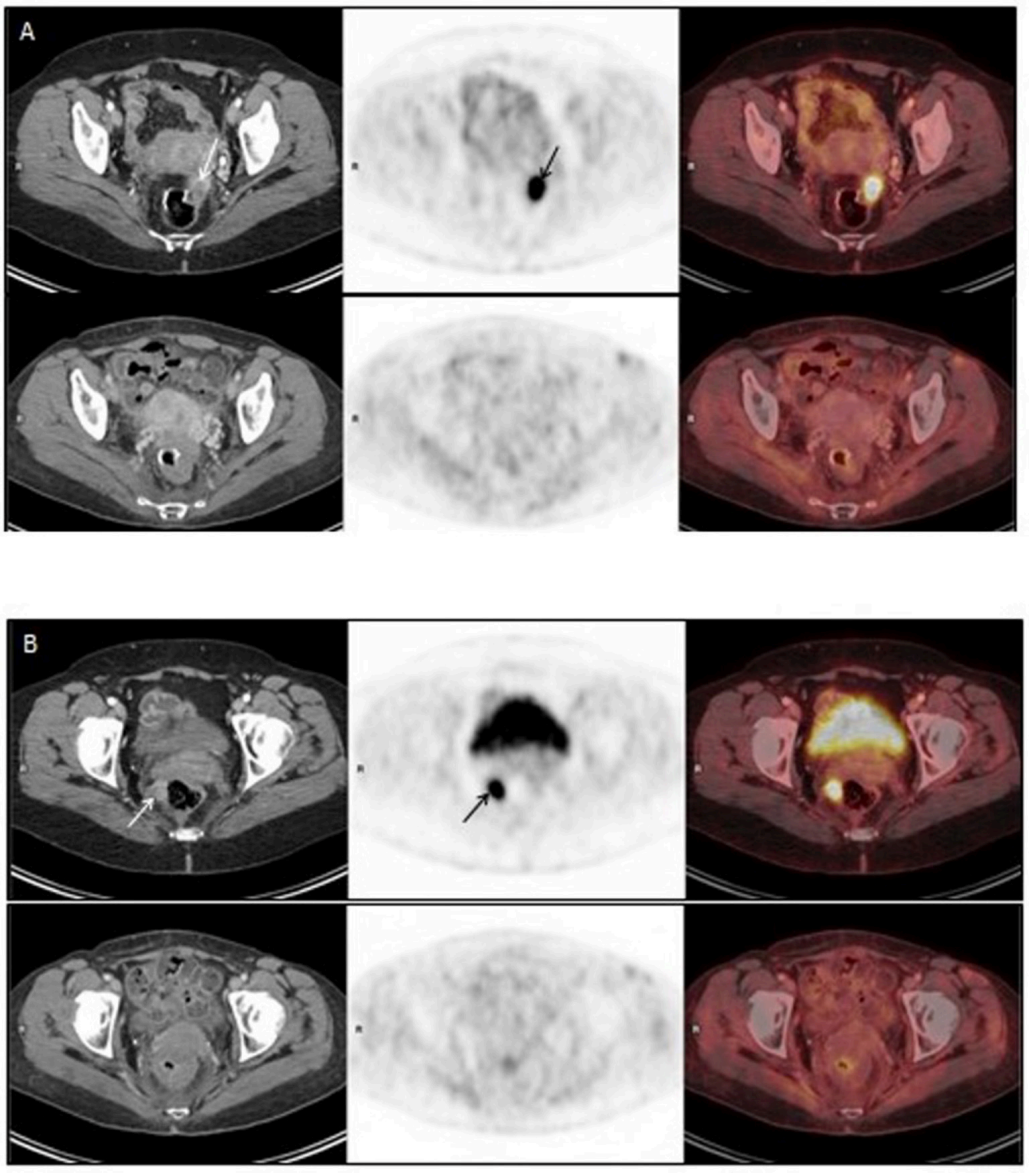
(A,B) represent PET-CT images of two different pelvic metastases in the radiation field. Upper panel of (A,B): PET/CT on 30Sep2015, before Promitil with radiotherapy. Lower panel of (A,B): PET/CT on 28Dec2016, 15 months after Promitil with radiotherapy. Axial CT (left panel), FDG PET (middle panel) and fused FDG PET/CT (right panel) images of two metastatic lesions. The intense pathological uptake in a left nodule anteriorly to the rectal anastomosis (A, arrows) and in a right para-rectal nodule (B, arrows) has completely resolved with therapy on both PET and CT, along a prolonged follow-up (A,B, lower panel). Note post radiation rectal wall thickening on post-therapy CT images.
